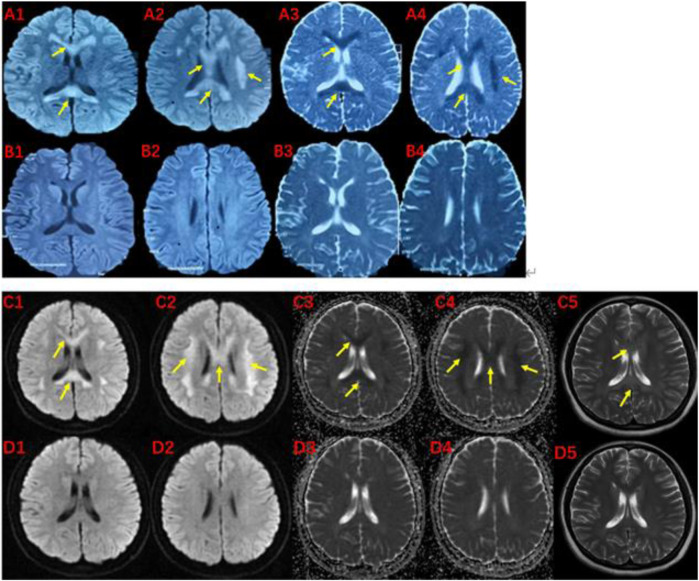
MRI of the proband (III-1) before and after the onset of the disease at 19 (A, B) and 27 (C, D) years of age.The brain MRI showed high intensities in bilateral white matter in the centrum semiovale and corpus callosum on diffusion-weighted (A1,A2,C1,C2), T2-weighted (C5), and low intensities on apparent diffusion coefficient images (A3,A4,C3,C4). These abnormal intensities disappear within two weeks (B1-B4 and D1-D5). The yellow arrow indicates involved structures.
